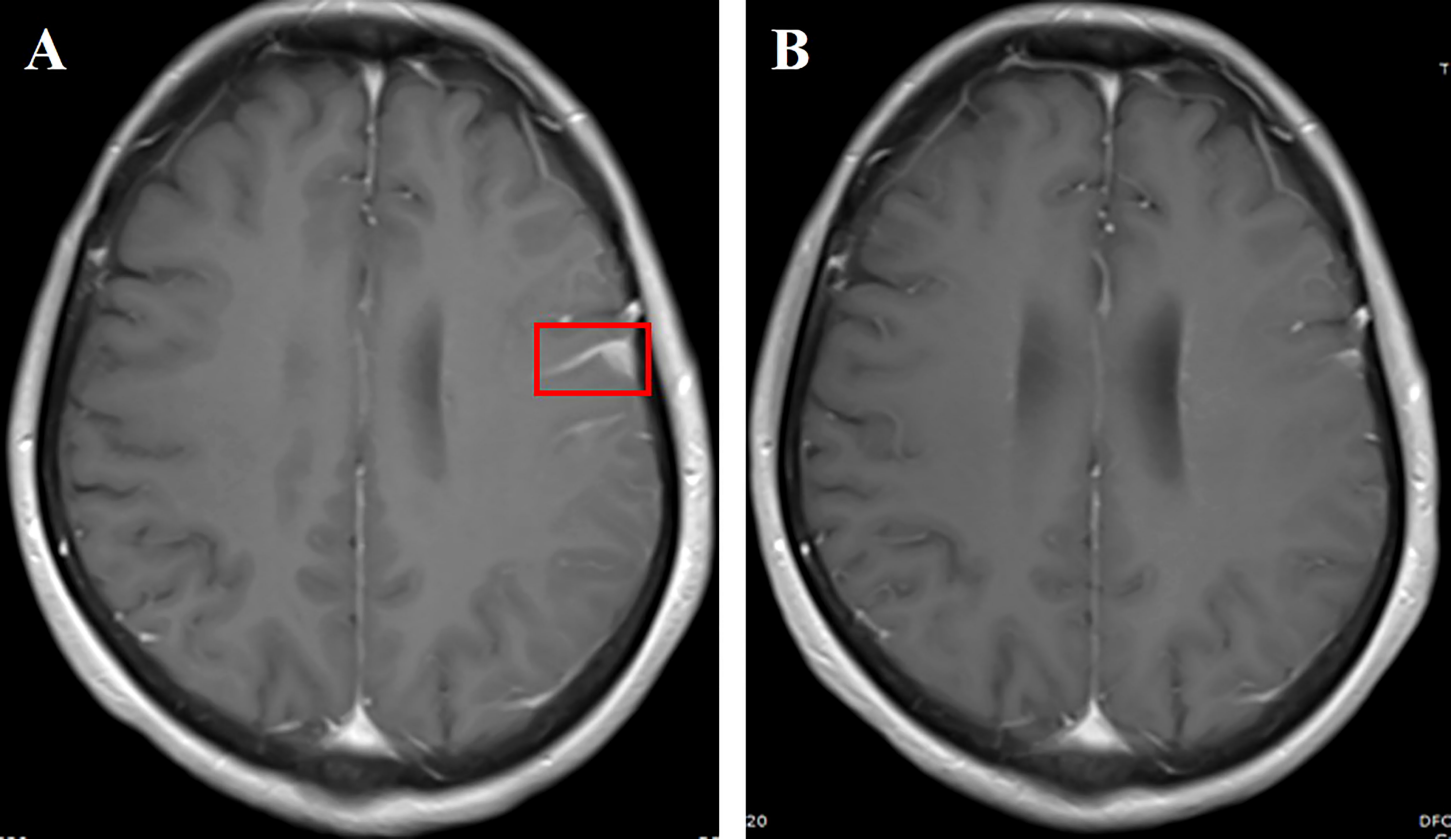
(A) Cranial enhanced MRI showing left frontotemporal linear enhancement (red box); (B) linear enhancement of leptomeninges shrunk 2 months after aumolertinib plus bevacizumab treatment.
